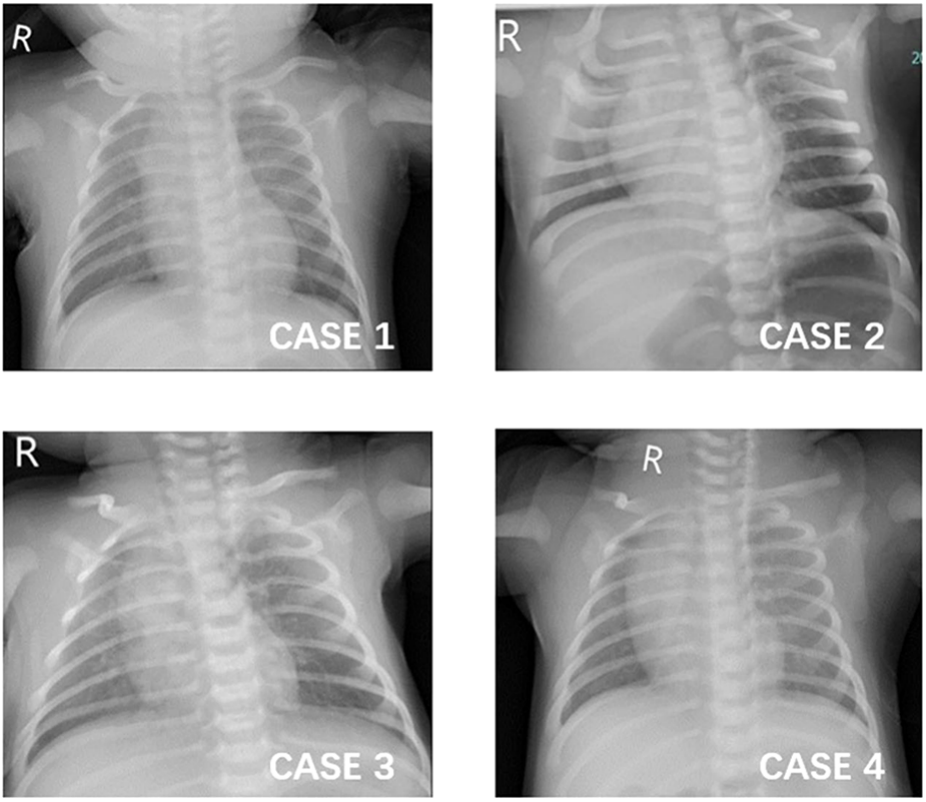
Chest x-ray images of the four patients. (1) CASE 1: increased and blurred bilateral lung markings. (2) CASE 2: increased and blurred bilateral lung markings. (3) CASE 3: increased and blurred bilateral lung markings and patchy shadows on the right upper lung field. (4) CASE 4: increased and blurred bilateral lung markings.
